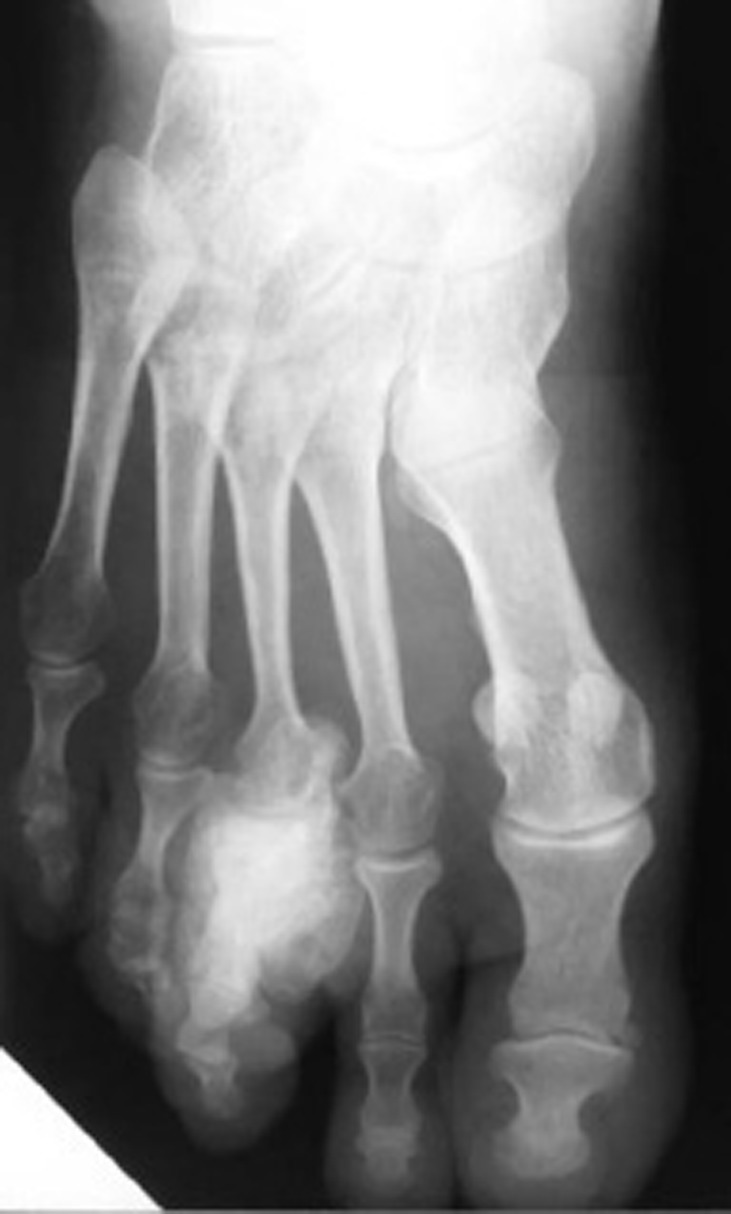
A 38-year-old woman with swelling and discomfort of the right forefoot. Antero posterior radiograph of the right foot shows an ossified mass originated from the proximal phalanx of the third toe.
